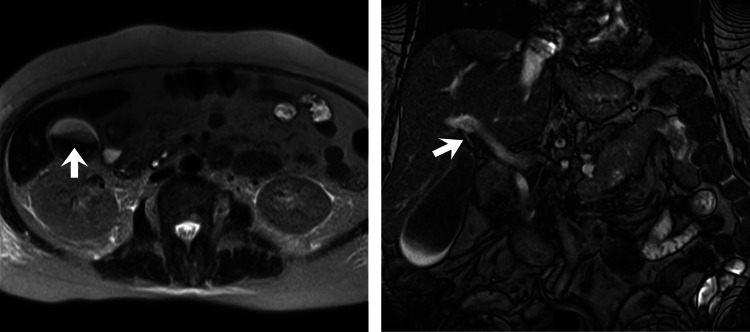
Contrast-enhanced abdominal magnetic resonance imaging. Gallbladder sludge: represented by an arrowhead in the image. Mild dilatation of the extrahepatic bile duct: indicated by a white arrow in the image.
